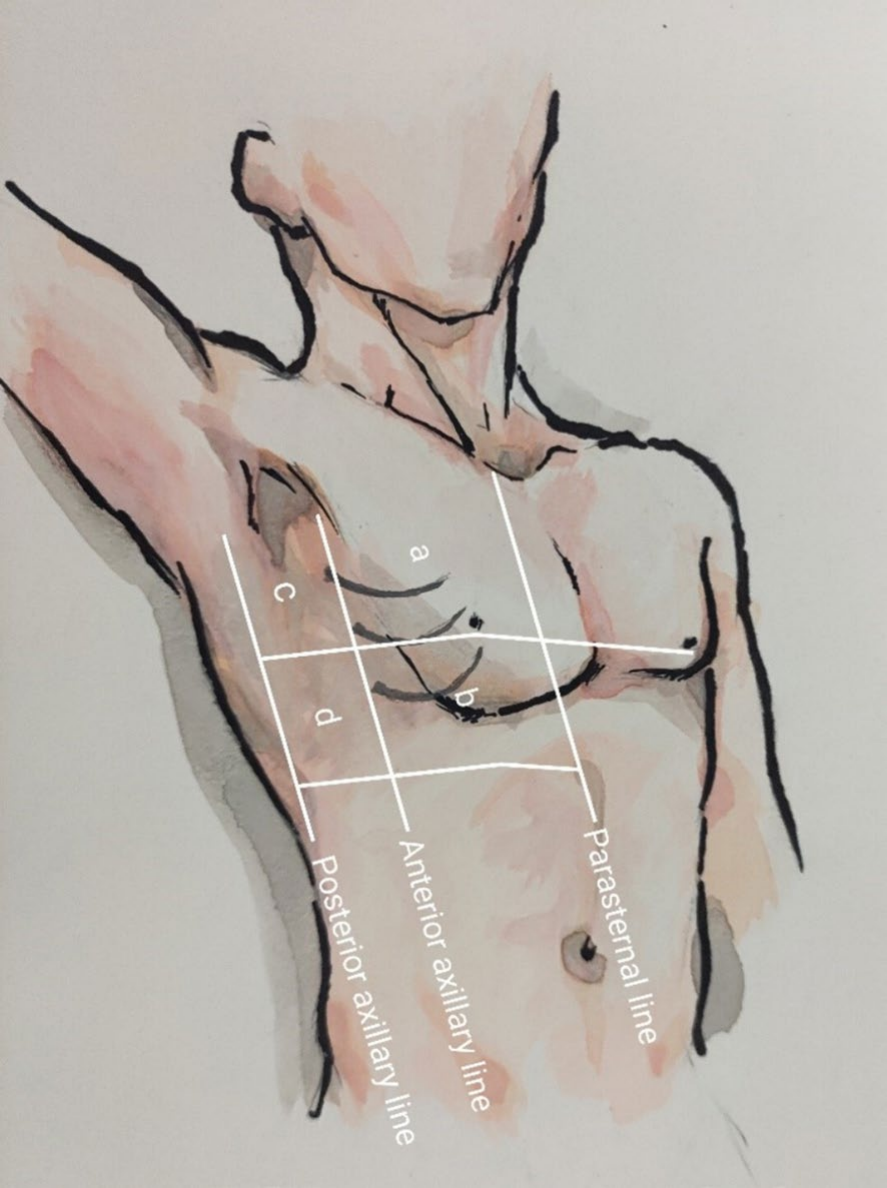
Zones followed for lung ultrasound examination
